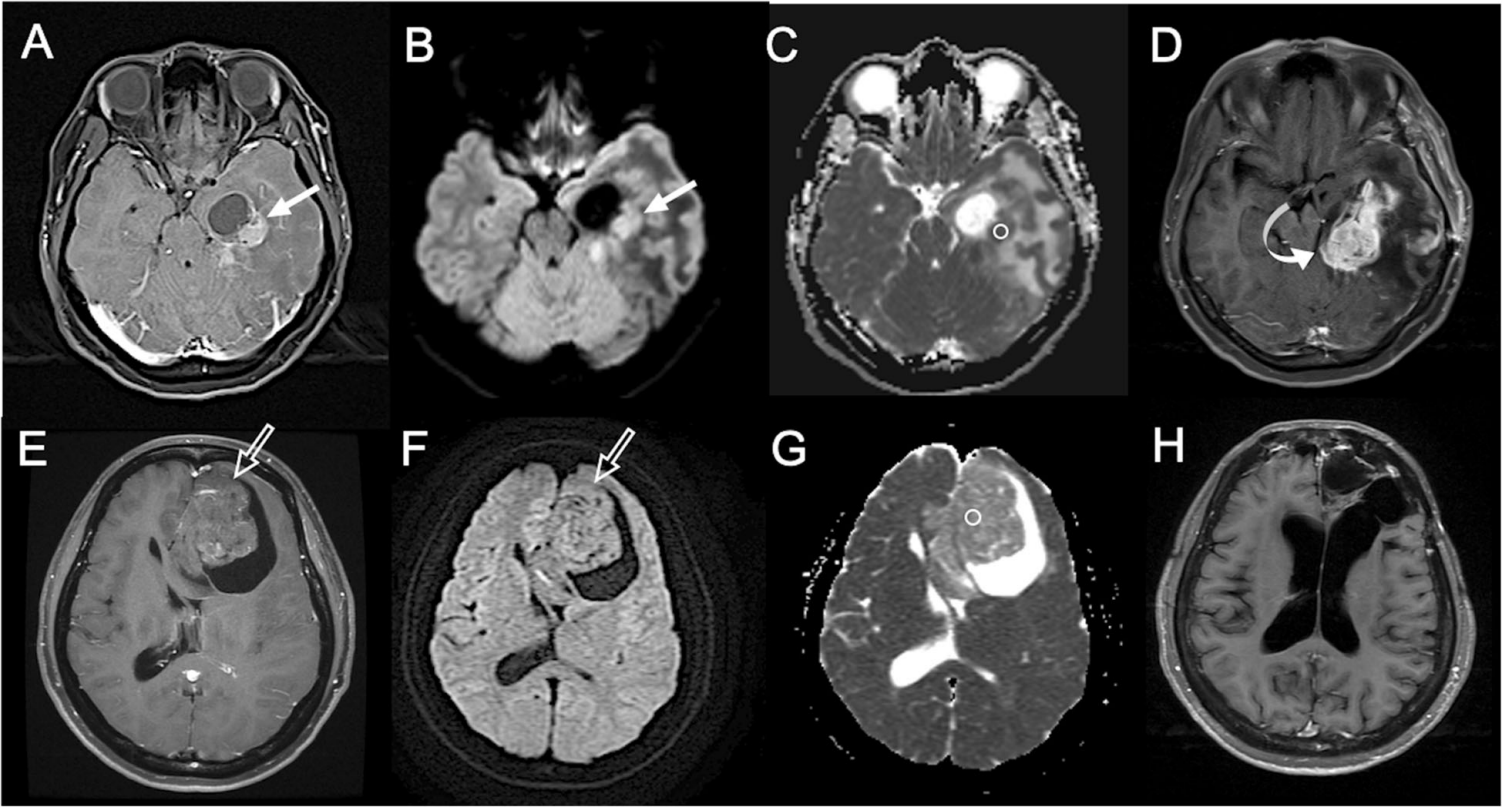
Low apparent diffusion coefficient (ADC) value on diffusion-weighted imaging (DWI) can predict worse therapeutic response in brain glioblastoma. A Pretreatment CE axial T1-weighted imaging (T1WI) shows a 40-mm-sized enhancing glioblastoma (arrow) with the cystic component in the left temporal lobe. B The DWI shows hyperintensity in the solid part of the tumor (arrow), indicating a diffusion restriction phenomenon. C The measured ADC value (b = 1000 s/mm^2^) on ROI is 0.72 × 10^− 3^ mm^2^/sec. (D) Rapid tumor recurrence (tumor diameter of 54 mm) (curved arrow) was observed 3 months after surgical resection. E Pretreatment CE axial T1WI shows another 65-mm-sized enhancing glioblastoma (open arrow) with a cystic component in the left frontal lobe. F DWI shows isointensity (no diffusion restriction) in the solid part of the tumor (open arrow). G The measured ADC value on ROI is 1.42 × 10^− 3^ mm^2^/sec. (H) No tumor recurrence was observed 72 months after surgical resection
